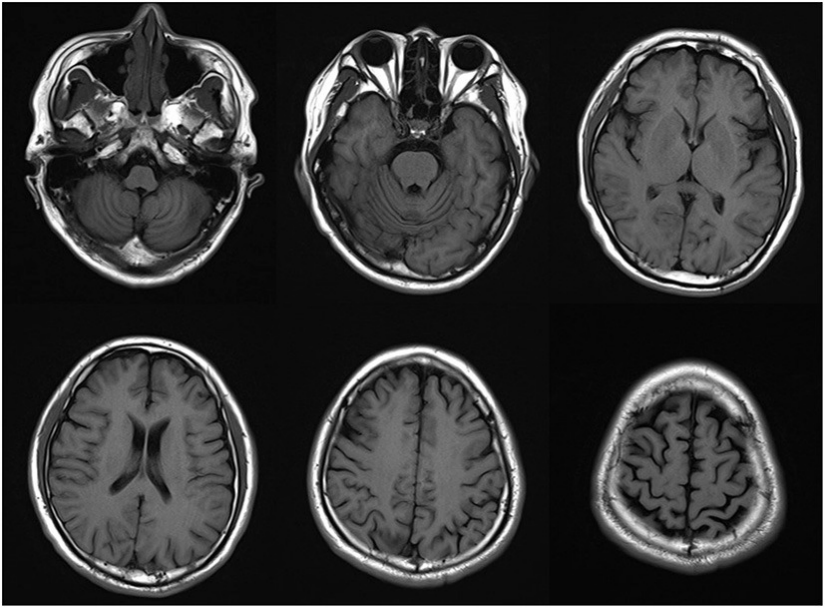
Brain MRI showed atrophy of the cerebrum and cerebellum.
